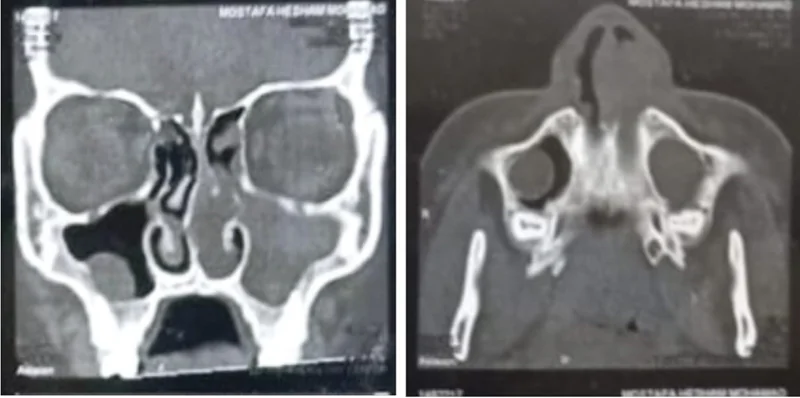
Axial and coronal CT nose and PNS showing left ACP and right antral cyst.
